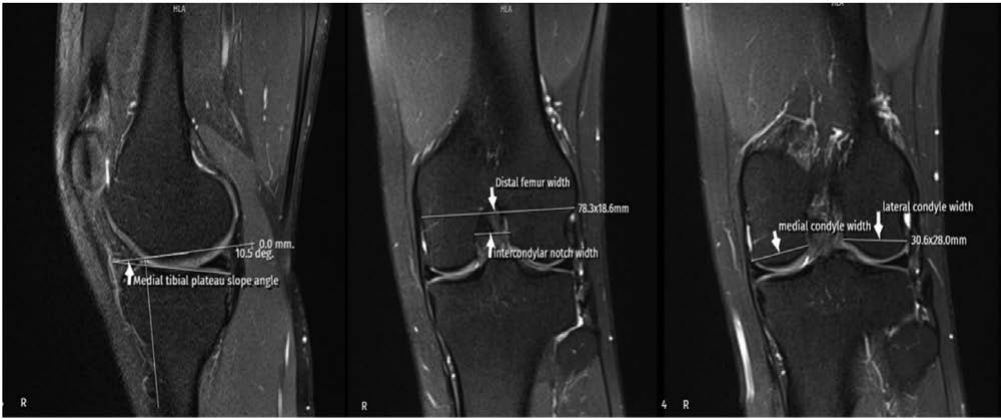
MRI measurement techniques of MTPS, IFW, MFCW, and LFCW. IFW = intercondylar femoral width, LFCW = lateral femoral condylar width, MFCW = medial femoral condylar width, MRI = magnetic resonance imaging, MTPS = medial tibial plateau slope.
